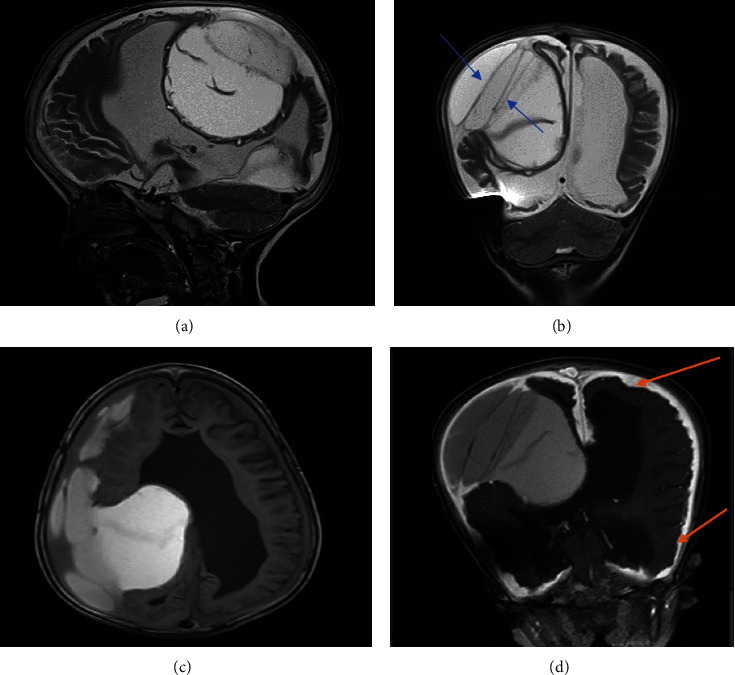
Sagittal T2W (a) and coronal T2W images demonstrated right pariental complex fluid collection with internal septation (blue arrow) and different internal hemorrhagic stages and mass effect (b). Axial FLAIR image demonstrated that collection over right temporoparietal extraaxial collection with mass effect has focal internal layering (c). Contrast-enhanced T1W image showed diffuse nodular dural thickening and dural enhancement (orange arrow) (d).
